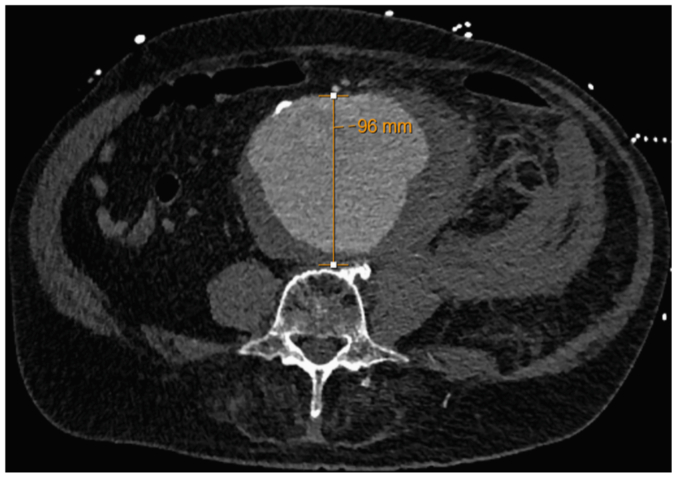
Computed tomography angiography (CTA) of the aneurysm rupture. Displayed is a maximal diameter of 96 mm. The retroperitoneal hematoma is visible in the left flank.
